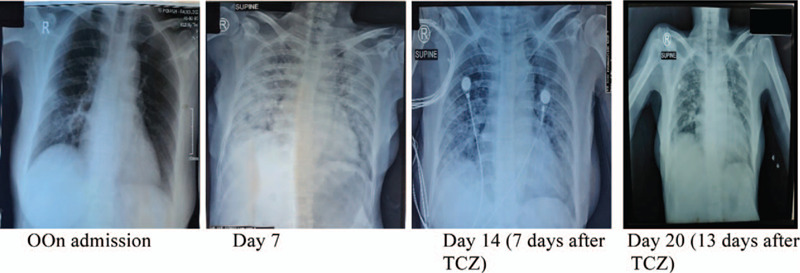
Serial chest x-rays. TCZ was administered at day 7 (when ARDS is confirmed).
